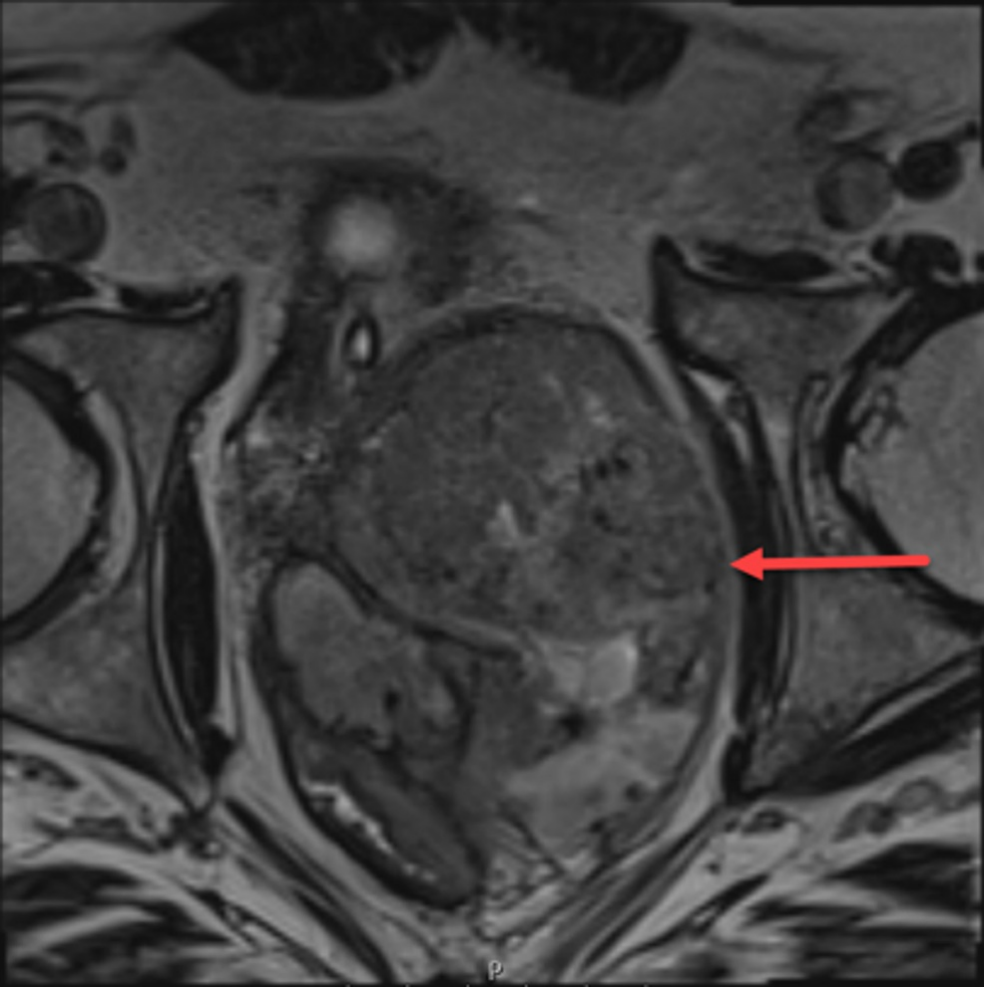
Axial MRI T2 showing heterogeneous necrotic mass arising from the left prostate (red arrow), invading the rectal wall
